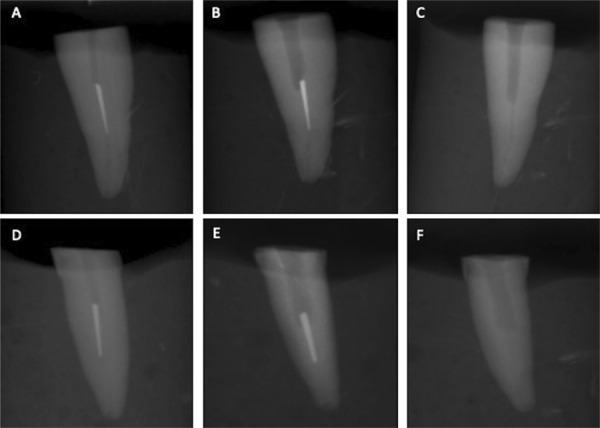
Representative periapical radiographs of the experimental procedures. After an instrument fracture was created (A), access to the instrument was obtained, a partial platform was prepared (B), and the instrument was removed with ultrasonic tips (C). After an instrument fracture was created (D), access to the instrument was obtained, a full platform was prepared (E), and the instrument was removed with a BTR Pen (F).
